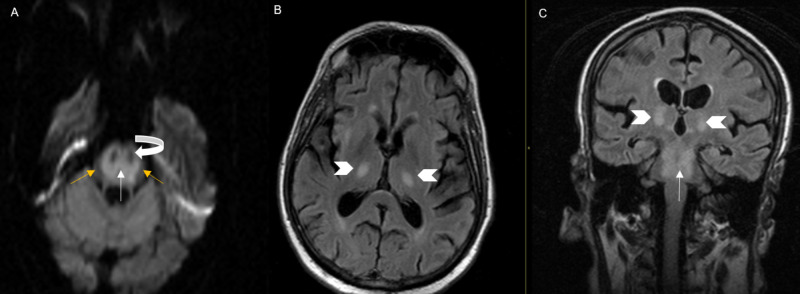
Axial diffusion-weighted (DWI) magnetic resonance imaging (MRI) (A) MRI demonstrates diffusion restriction involving the central pons (white arrow), sparing the periphery (yellow arrow), as well as corticospinal tract (curved white arrow). (B) Axial and (C) coronal flair images showing extra pontine involvement with T2 prolongation involving the bilateral pons (arrowhead) in addition to the central pons (white arrow).
